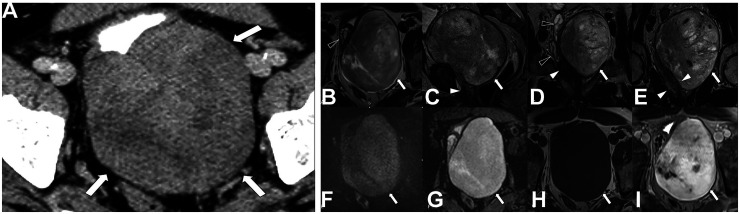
Radiologic evaluation of the pelvic mass. (A) Axial post contrast computed tomography shows a large heterogeneously enhancing mass (white arrows) superior to the prostate and bladder. (B-I) Magnetic resonance imaging of the pelvis with and without contrast (B—axial T2, C—sagittal T2, D and E—coronal T2, F—axial diffusion—weighted imaging, G—apparent diffusion coefficient, H—axial T1, I—axial T1 post contrast) demonstrates a large heterogenous T2 (B-E) intermediate mass (white arrows) superior to the left of the prostate (white arrowheads) with marked mass effect on the prostate and the seminal vesicles (black arrowheads), which are deviated anteriorly to the right. This mass demonstrates no restricted diffusion (F and G), is T1 hypointense (H) with heterogeneous enhancement (I).
